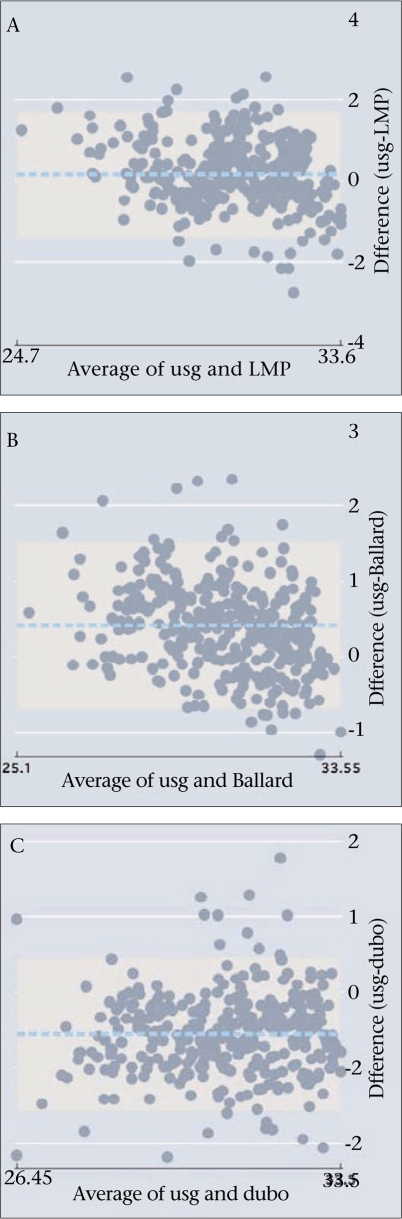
Bland-Altman plots of prenatal ultrasound GA (usg) estimates with (A) LMP, (B) Ballard score, and (C) Dubowitz (dubo) score, in weeks
